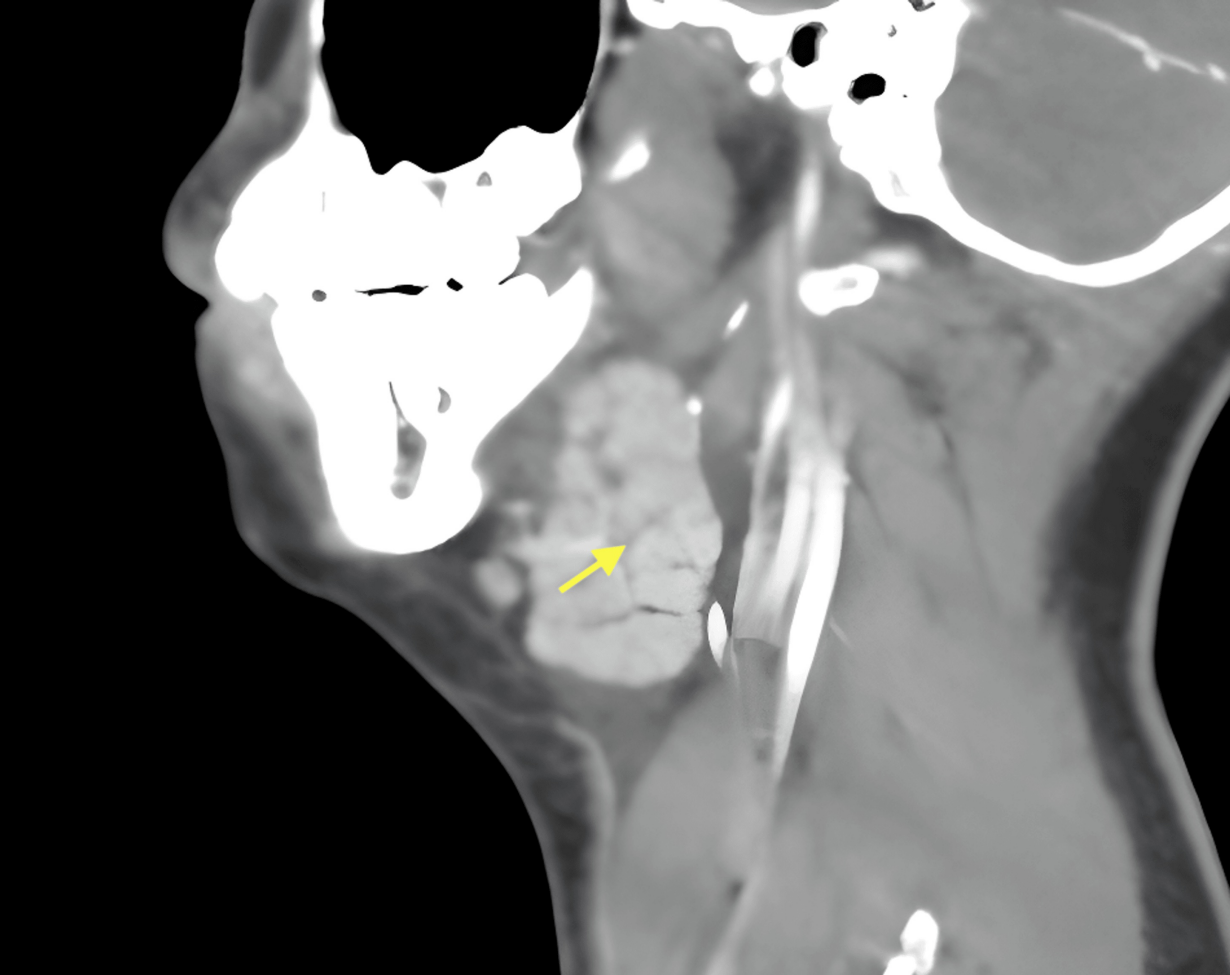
Sagittal CT neck with contrast Yellow arrow indicates the inflamed submandibular gland.
